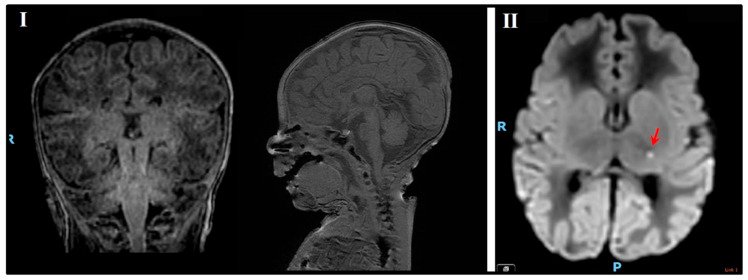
Postmortem MRI brain scan of male infant described in Case A. (I) T1 weighted imaging displaying absence of neuroanatomic defects. (II) Diffusion weighted imaging showing mild ventricular bleed, a thalamic infarct (Lt small) located in the left internal capsule as indicated by the red arrow. R = Right; P = Posterior.
